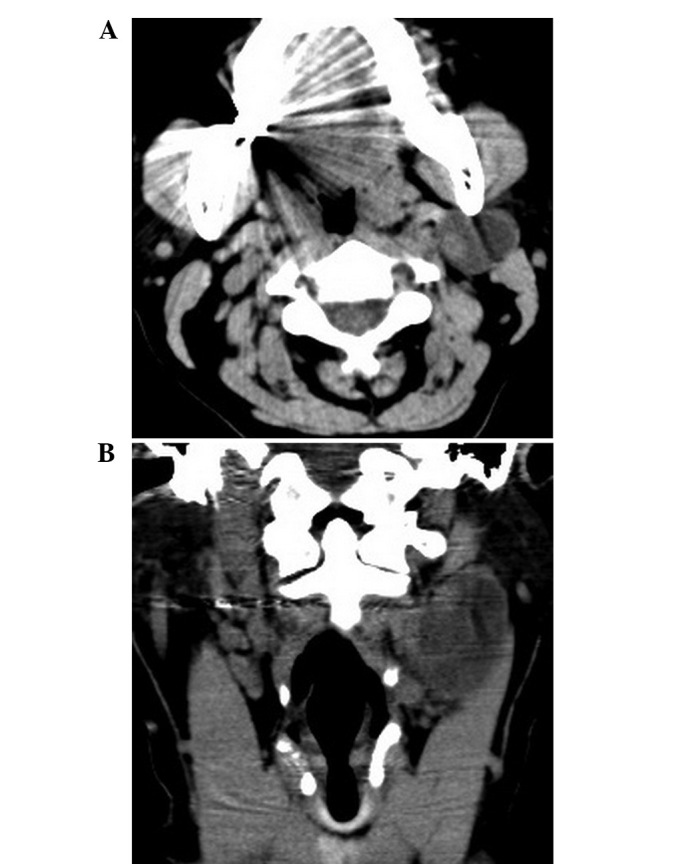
Type II BCC with malignant transformation. (A) A well-defined cystic mass with an intralumenal node was observed on CT scan. (B) In coronal planes, a node located in the inner wall of BCC was observed. BCC, branchial cleft cyst; CT, computerized tomography.
